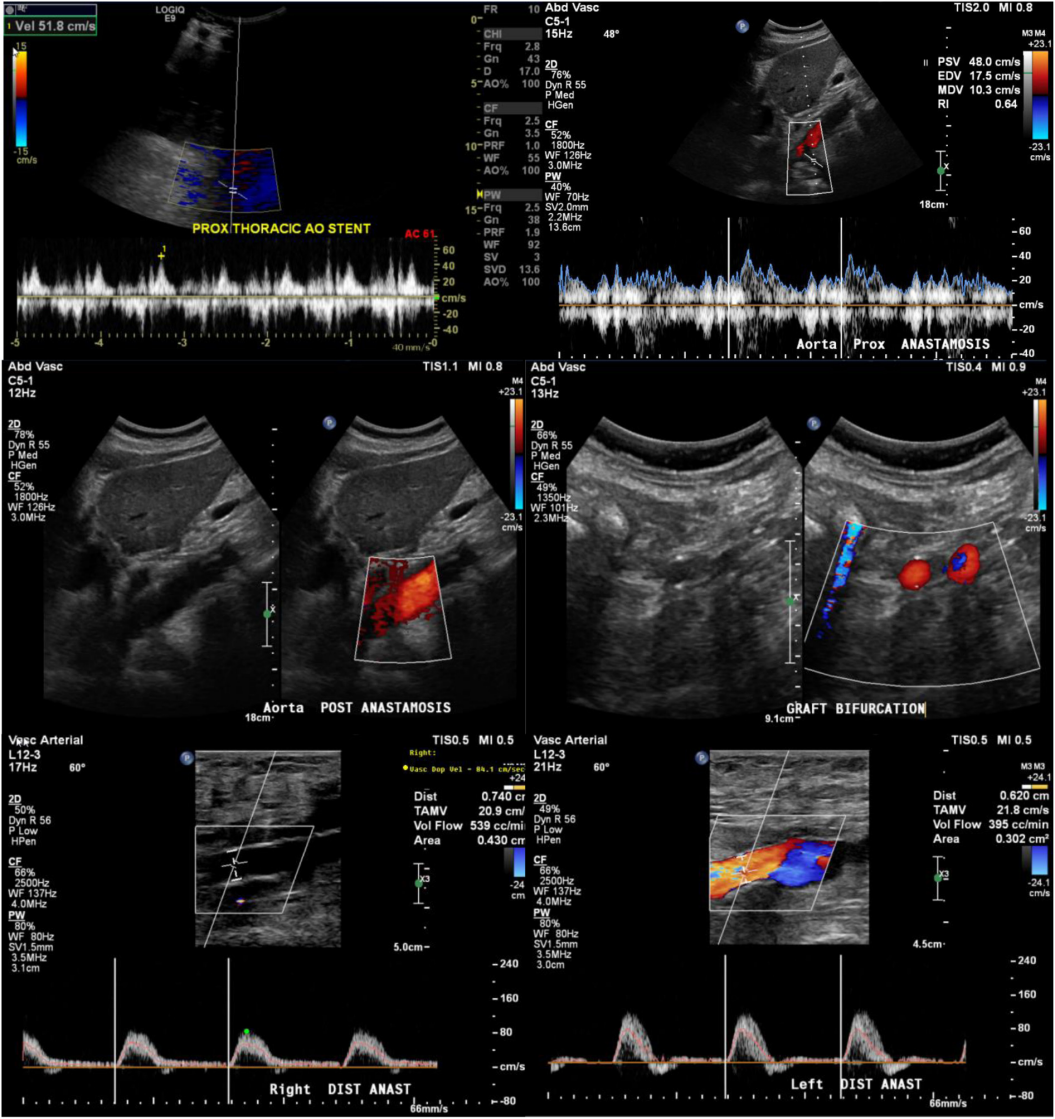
Mid-term (5-month) follow-up duplex ultrasound images showing patent descending thoracic aorta to bilateral femoral artery bypass (DTFB) and thoracic endovascular aortic repair (TEVAR). *ANAST,* anastomosis; *DIST,* distal; *PROX,* proximal.
